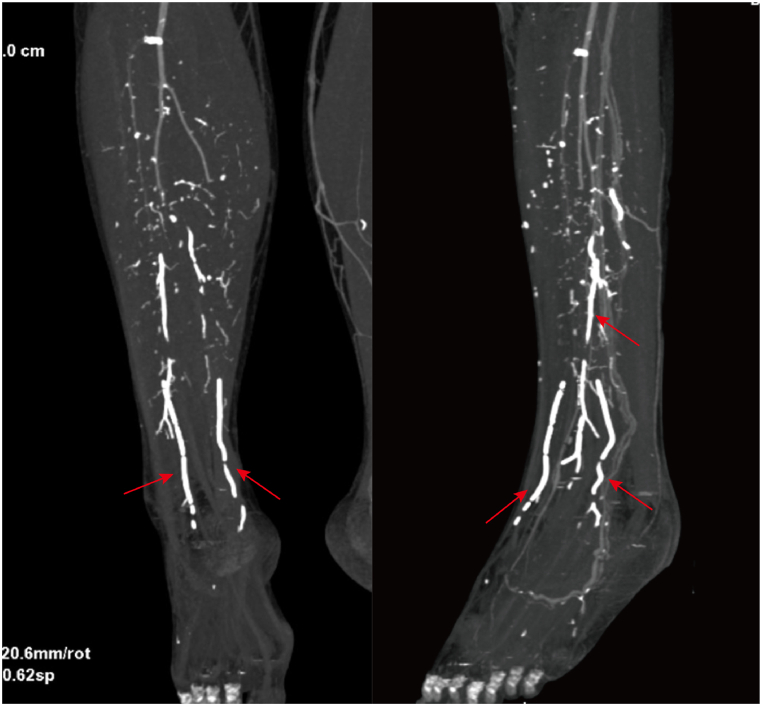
The CT angiography of the arteries of the lower extremities showed a linear, dense signal within the anterior tibial artery, posterior tibial artery, and peroneal artery, which was initially considered to be the results of cement embolization of the arteries (red arrows).
